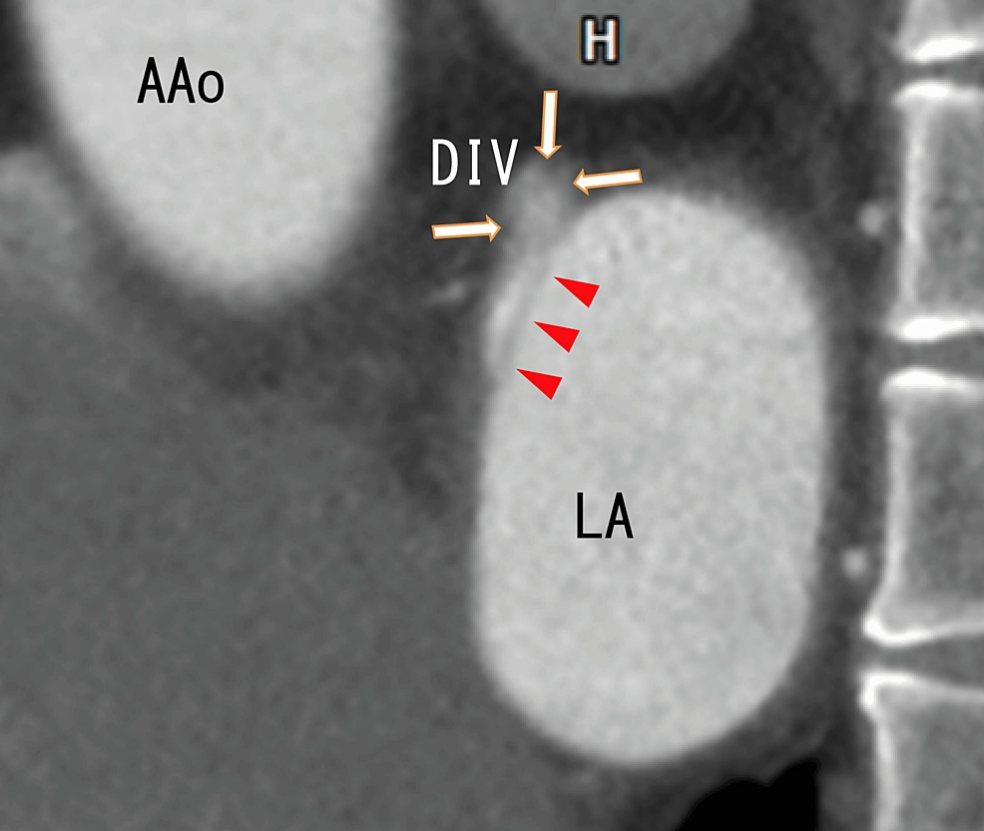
Sagittal images from an 80-MDCT scan revealing the attachment region in the LA and LAD Sagittal images from an 80-MDCT scan reveal an LAD and LA. There were no images of thrombi in the LA. There was a dark line near the anterosuperior wall of the LA (arrowheads), suggesting that the LA thrombi were attached to the anterosuperior wall of the LA. At the top of the line, there was an LAD, the border of which was vaguely depicted. 80-MDCT, 80-slice multidetector computed tomography; AAo, ascending aorta; LA, left atrium; DIV, diverticulum.
